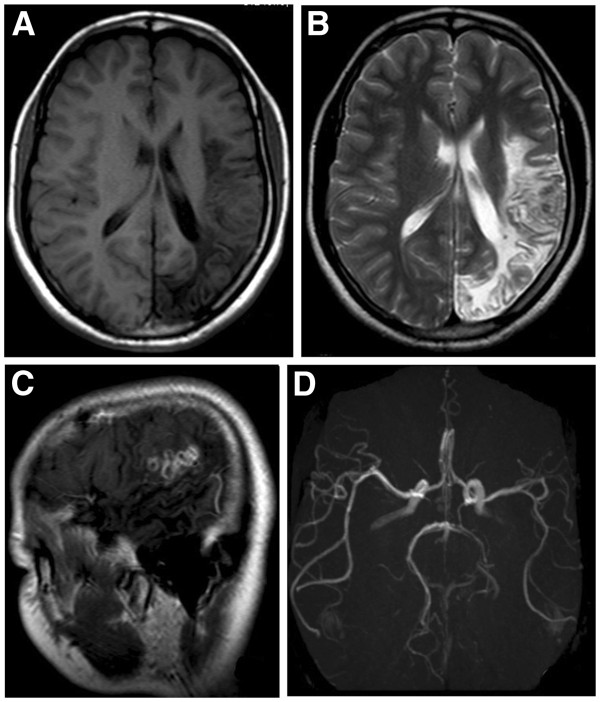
MRI images for the case 15 in the three-month follow-up. A. The T1W image shows there is an irregular large patchy area with hypointensity in the left parietal lobe including white matter, gray-white matter junction, and gray mater areas. Parenchymal atrophy was seen as decrease in the size of ipsilateral gyrus and sulcus, but increase in the size of corresponding fissures of the lesion and the left ventricle. B. The T2W image shows there is an irregular large patchy area with hyperintensity in the left parietal lobe including white matter, gray-white matter junction, and gray mater areas. Atrophy of parenchyma is seen as the same as seen in the T1W image. C. The enhanced T1W image shows a few beaded enhancing lesions. D. The MRA slice shows decrease in the size and number of the branches in the left posterior artery.
